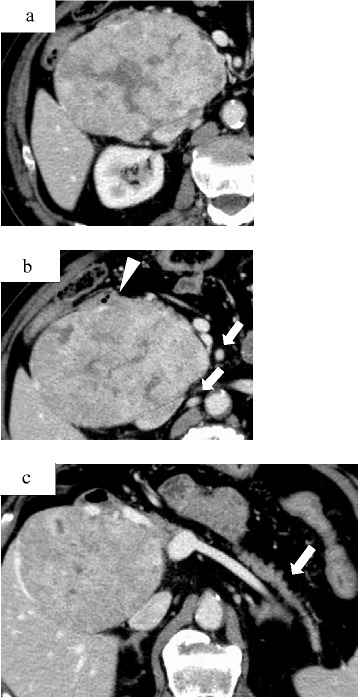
Computed tomography scan image demonstrating a heterogenous enhancing mass with areas of necrosis replacing the head of the pancreas (a). Computed tomography scan image demonstrating local invasion into the peripancreatic retroperitoneum and duodenum (*arrowhead*) and lymph node involvement (*arrow*) (b). No other hypervascular insulinomas were identified in the pancreatic body and tail (*arrow*) (c)
